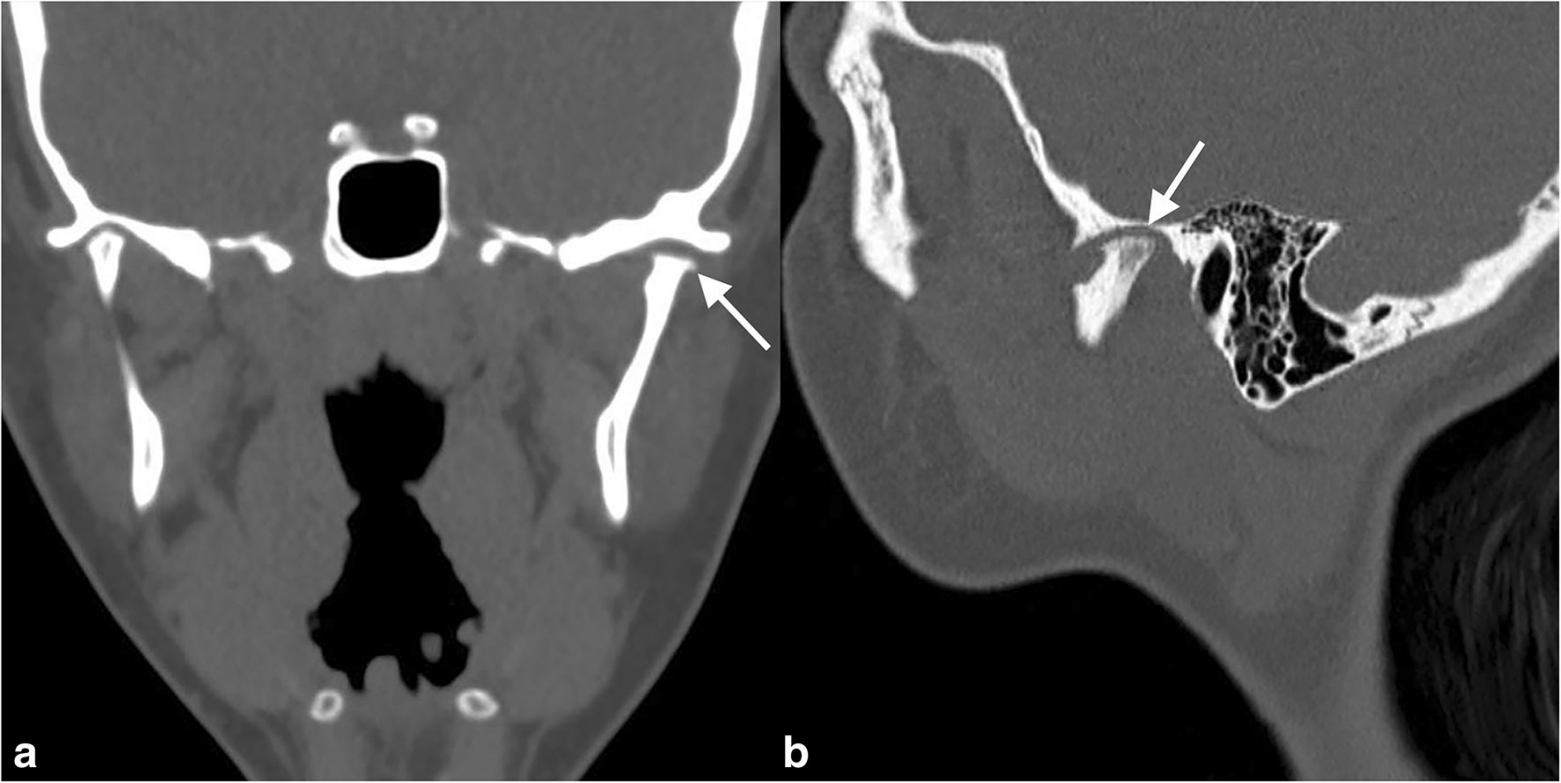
An 18-year-old girl with long-standing TMJ arthritis showing chronic condylar bony abnormalities of the left temporomandibular joint including flattening of the temporal fossa and the mandibular condyle (arrows) on (a) coronal and (b) sagittal CT images
